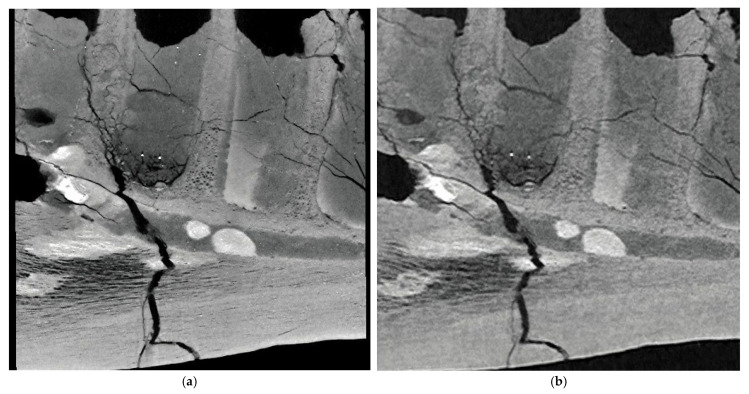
Sagittal sections of the *Tyrannosaurus* dentary bone imaged with PCD-CT (a) and EID-CT (b).
